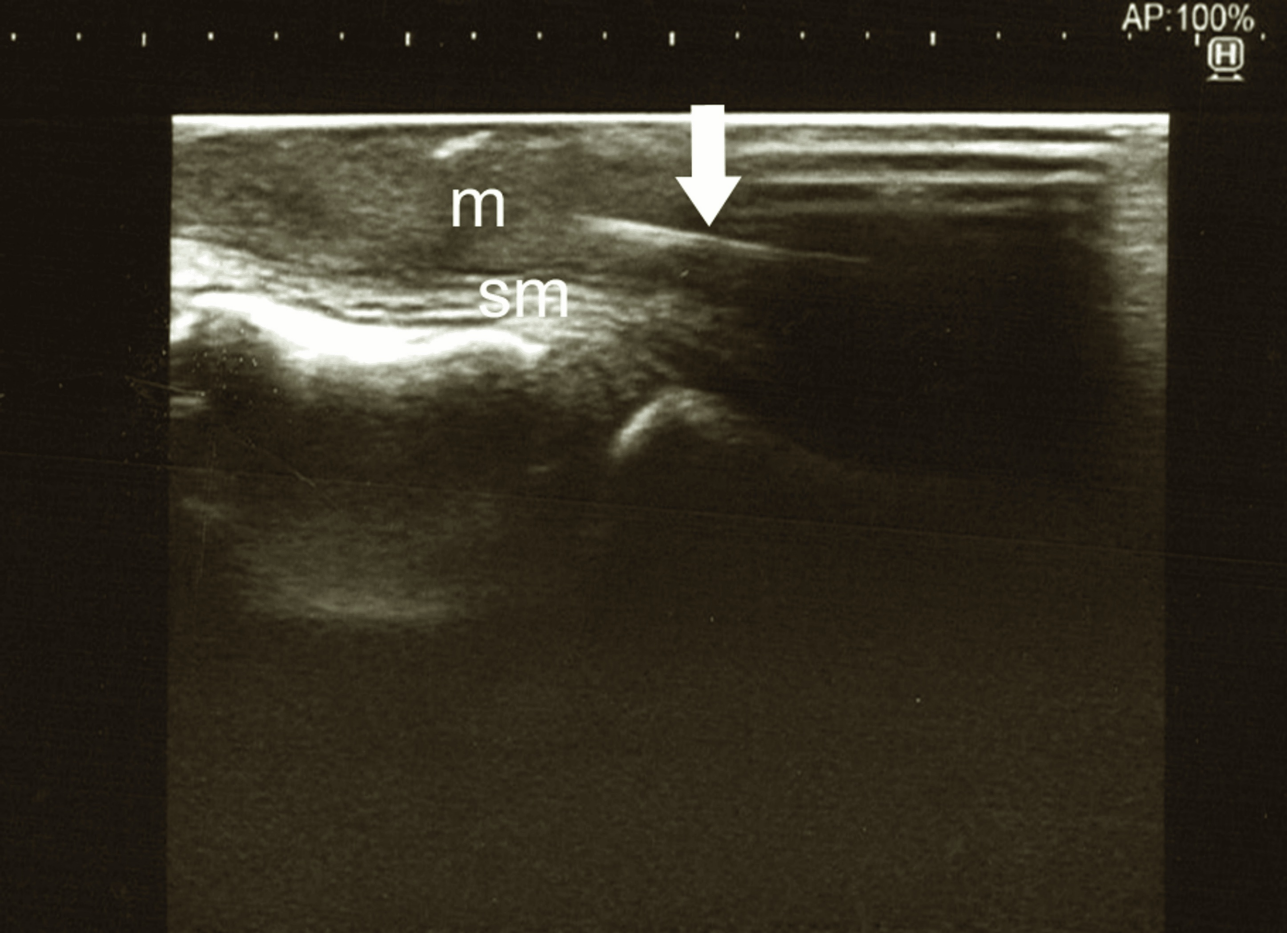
An ultrasound examination using a 13–6 MHz linear probe (Sonosite SⅡ, FUJIFILM, Tokyo, Japan) revealed the fish bone (arrow) located within the muscular layer of the esophageal wall. sm, submucosal layer; m, muscular layer
